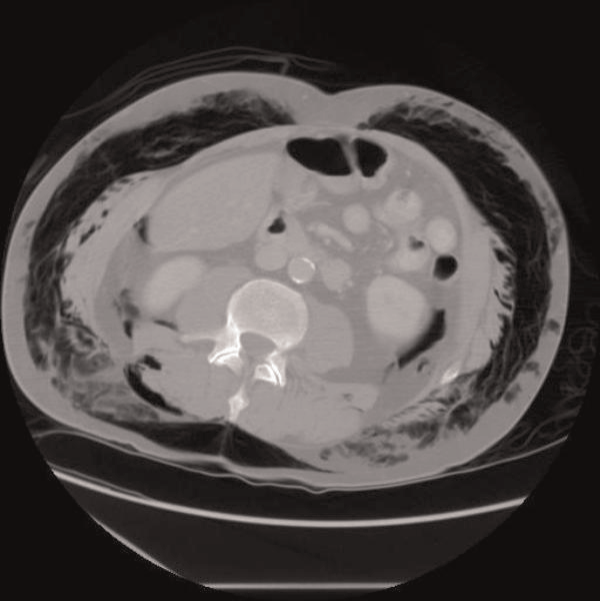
CT showing retropneumoperitoneum.
